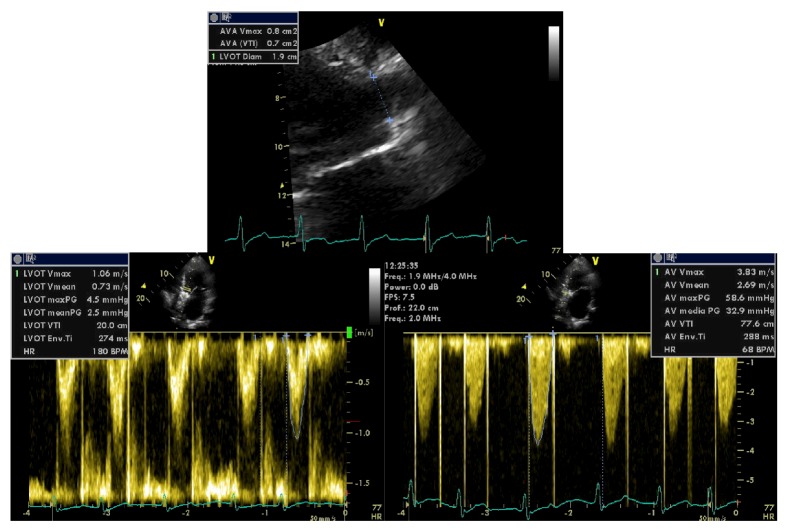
Effective orifice area calculation for the mechanical prosthesis on the transthoracic echocardiography. On the top, the left ventricle outflow tract (LVOT) diameter measurement; on the bottom, the pulse wave analysis on the LVOT on the left and the prosthesis continuous wave analysis on the right.
